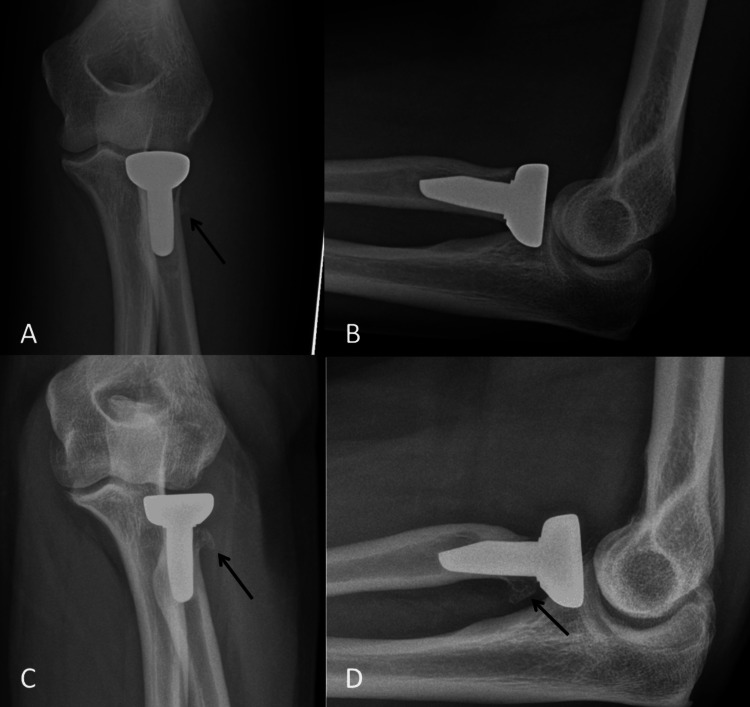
Post-operative radiographs taken at three weeks and six months after surgery. A: Antero-posterior view at three weeks after surgery demonstrating the subtle appearance of callus lateral to the level of the radial neck (black arrow). B: Lateral view showing a well-sized and well-fitting RHR. C: Antero-posterior view at six months after surgery showing mature callus at the radial neck (black arrow) and a degree of remodeling of the bone. D: Lateral view at six months after surgery showing the mature callus (black arrow) and a degree of loosening of the RHR. RHR, radial head replacement
